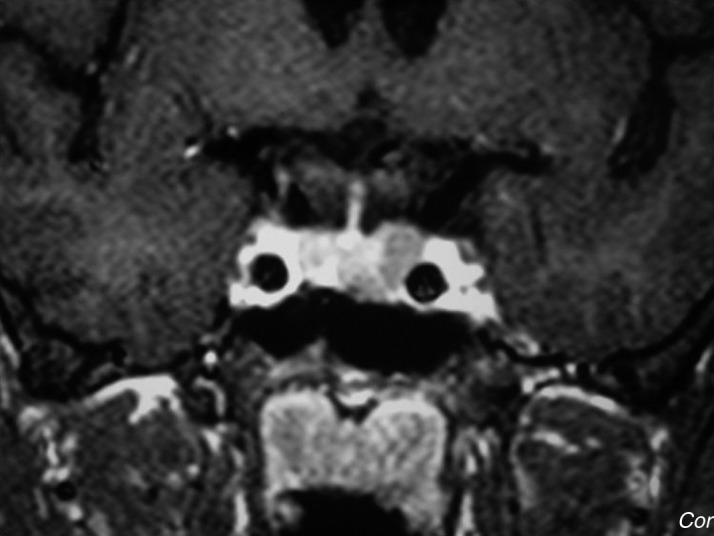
MRI sella showing left pituitary microadenoma.
